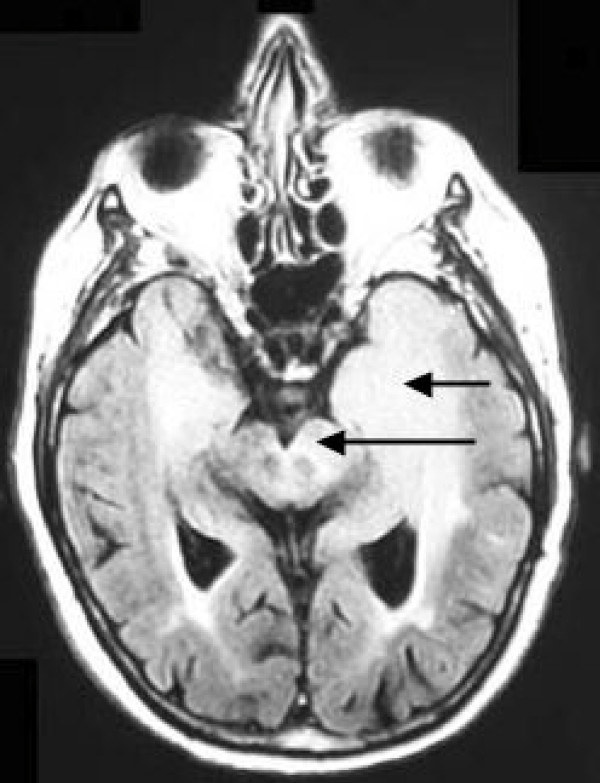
Axial fluid-attenuated inversion recovery MRI image demonstrating tumor-related infiltration involving both temporal lobes (Short arrow), and the substantia nigra (Long arrow).
